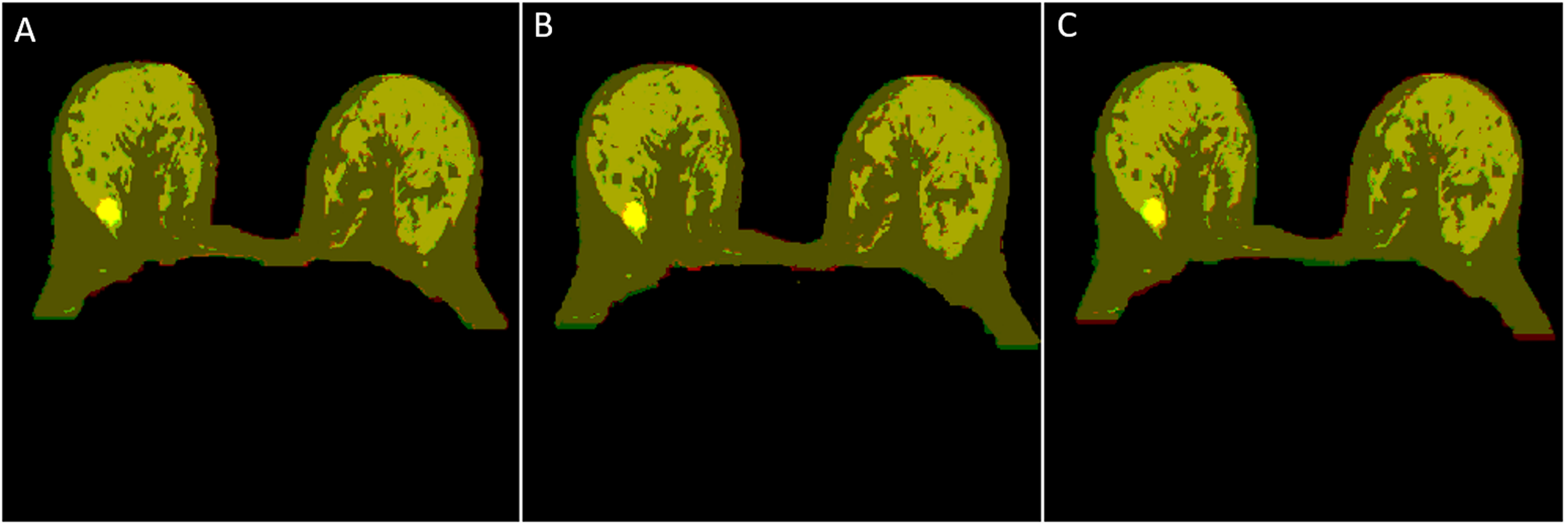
Comparison between different structural MRI images. (A), (B) and (C) show T_1_-W vs T_2_-W, PD-W vs T_2_-W and PD-W vs T_1_-W respectively. Yellow shades show overlapping between two mask whereas red and green color for present in one mask but not other mask respectively.
